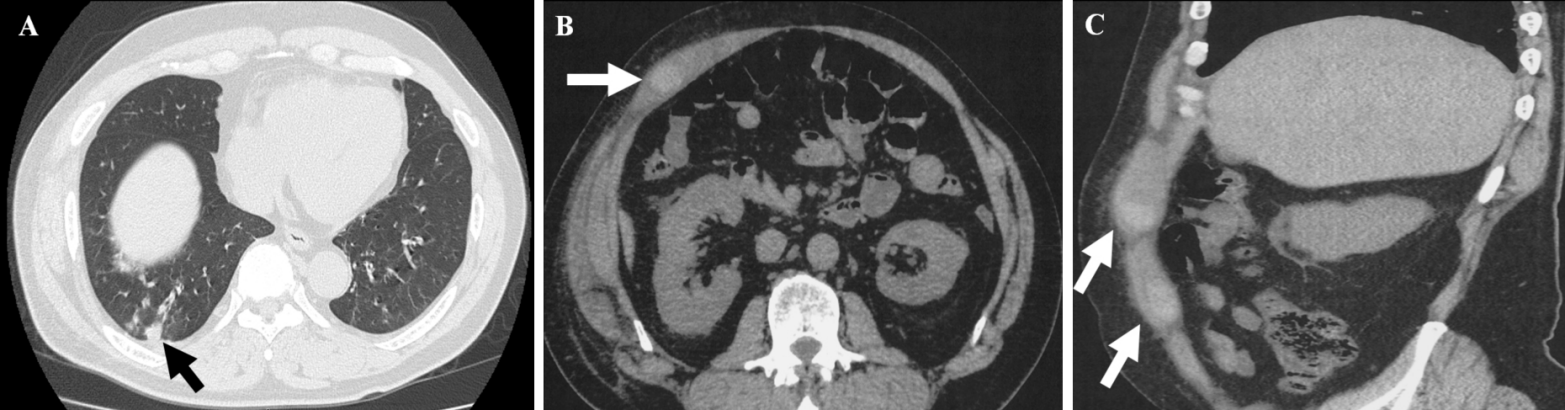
(A) Axial lung computed tomography (CT) scan showing right bronchopneumonia (arrow). (B) Axial abdominal CT scan showing hematomas (arrow). (C) Sagittal abdominal CT scan showing multiple hematomas (arrow).
